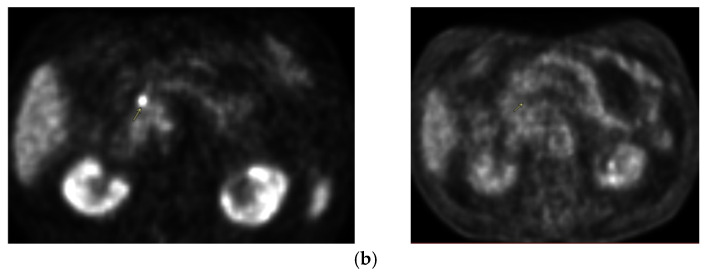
(a) Comparison between PET CT performed in 2018 (left scan) and November 2019 (right scan) without pathological radiotracer uptake foci corresponding to the location of the tumor lesion after SBRT. (b) Comparison between PET CT performed in 2018 (left scan) and November 2019 (right scan) without pathological radiotracer uptake foci corresponding to the location of the peripancreatic lymph node after SBRT.
